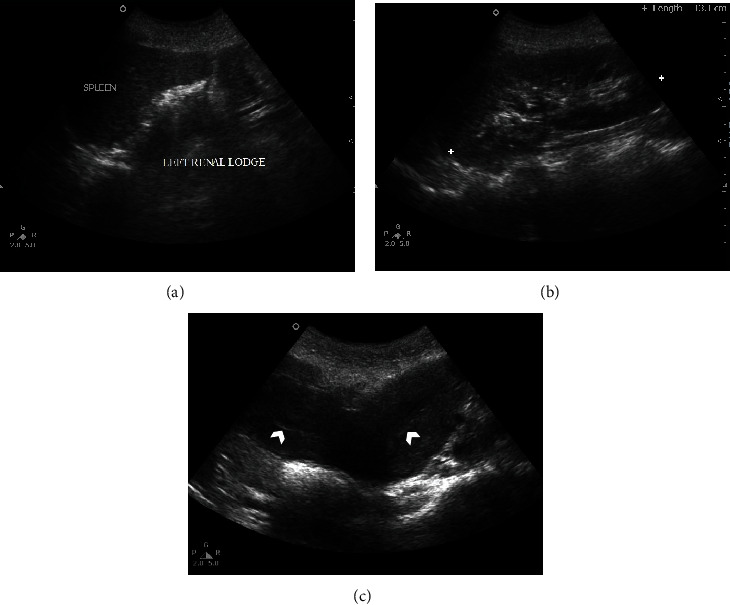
(a) Gray-scale ultrasound image of left renal lodge with nonvisualization of the left kidney. (b) Gray-scale ultrasound image of right renal lodge showing relatively compensatory prominent size of right kidney. (c) Gray-scale ultrasound image of the pelvis showing both uterine bodies. No dilatation of the endometrial canals (white arrowheads).
